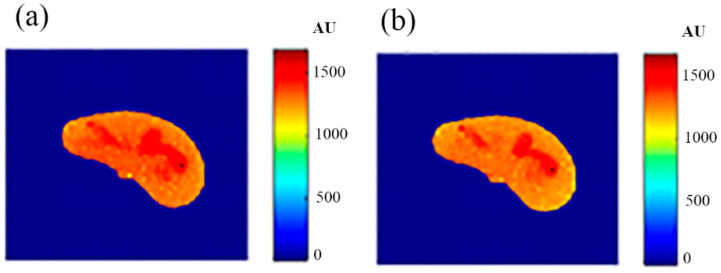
(a) The real MR image, and (b) the generated MR image using *T_E_* and *T_R_* of 25 ms and 2000 ms, respectively.
